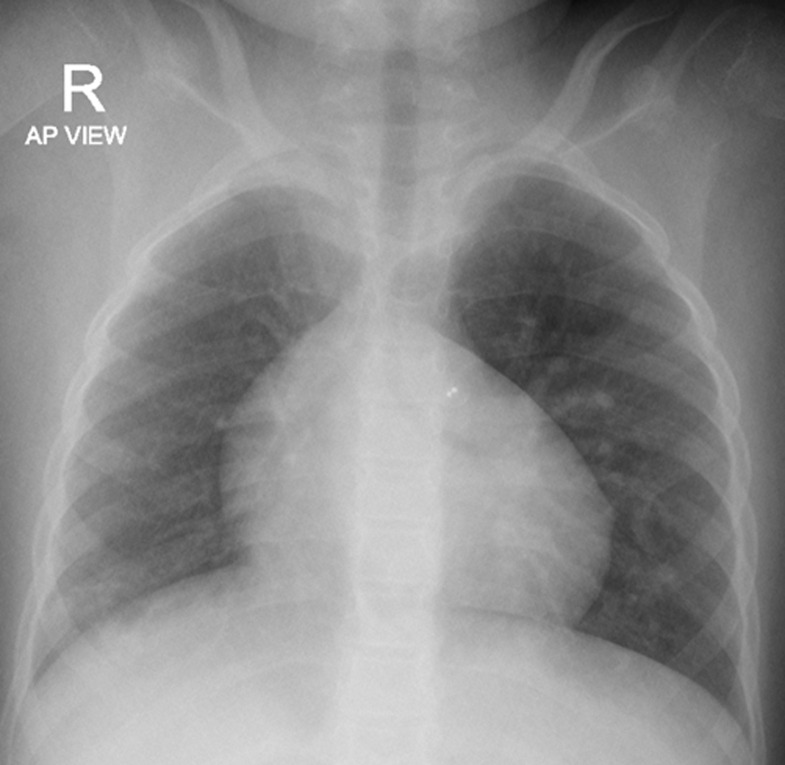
chest X-ray (antero-posterior view)
